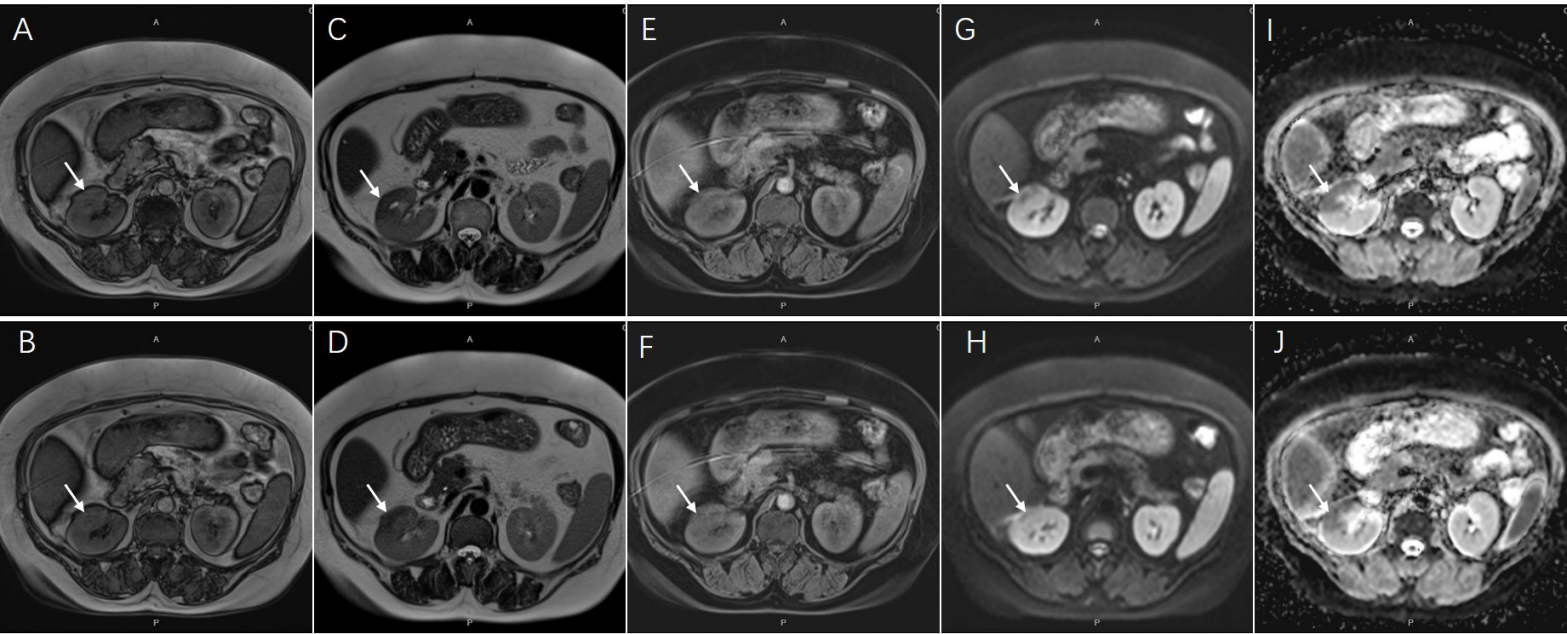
Magnetic resonance imaging showed an inhomogeneous lesion approximately 44x23mm in diameter [(A, B), coronal T1-weighted image; (C, D), coronal T2-weighted image, arrows]. The central area presented progressive enhancement in delayed phase [(E, F), arrows]. The lesion also showed an opposite signal found in center on diffusion-weighted sequences [(G, H), arrows]. The ADC map on corresponding areas presented low signal appearance [(I, J), arrows].
